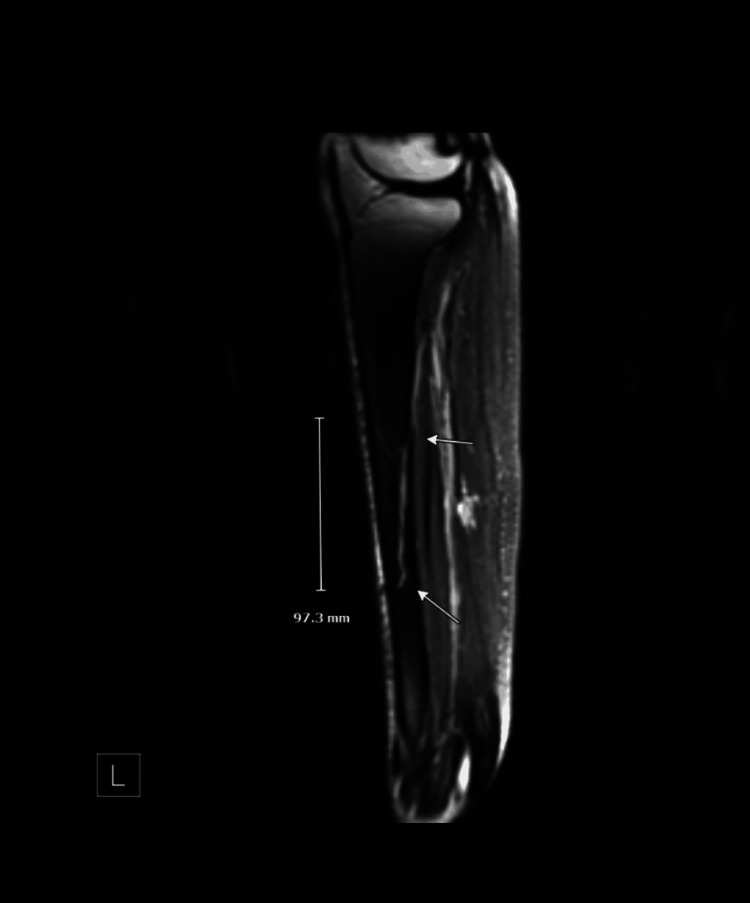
MRI of the left tibia and fibula, T2 sagittal view, revealing intraosseous varix in the central portion of the tibial diaphysis, with a craniocaudal extent of almost 10 cm
